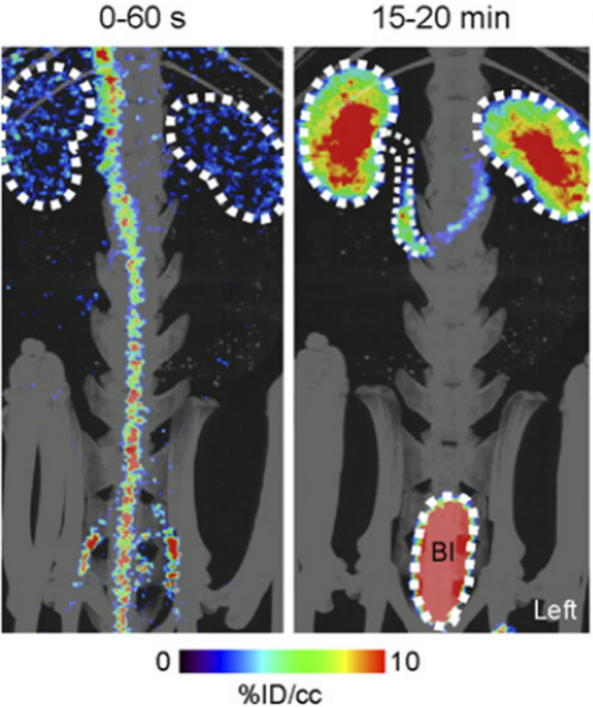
[^11^C]PABA PET/CT maximum-intensity-projection
